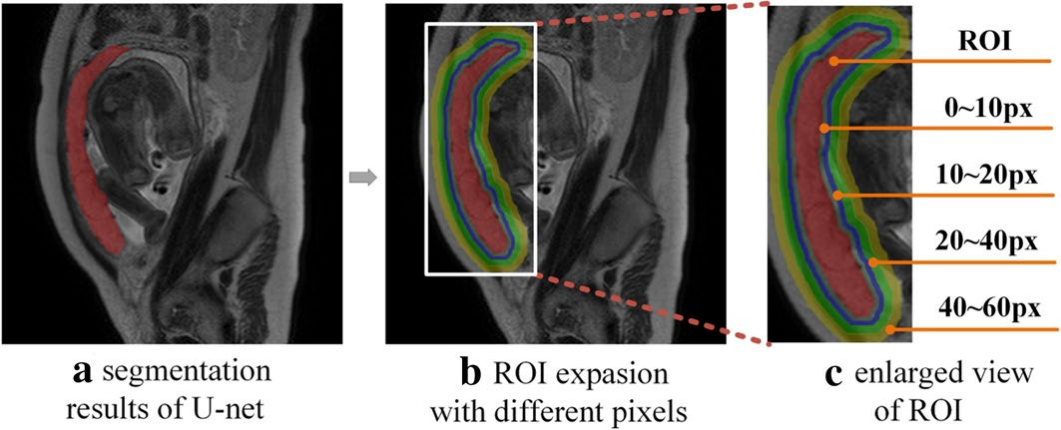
The ROIs formed with the different radial extension on T2WI MRI sagittal plane. The placental tissue is denoted as red, the colored circles denote different radial extensions of the placental tissue
